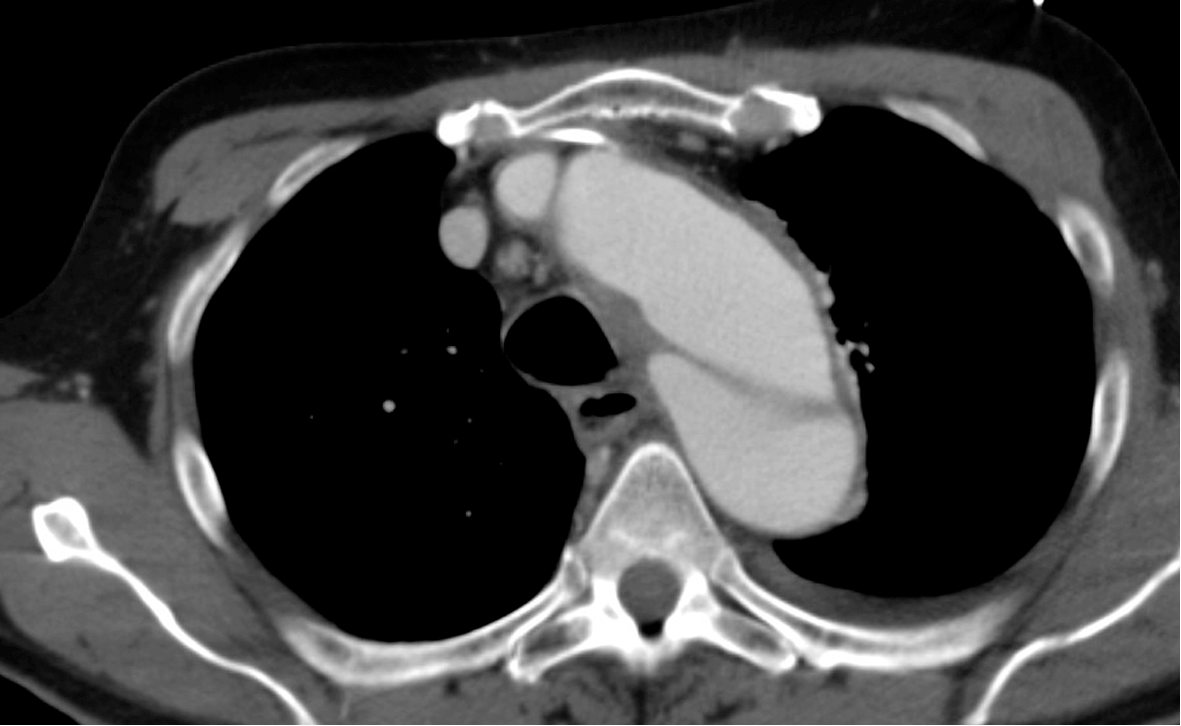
Thoracic aortic CT-scan at admission reveals a classic Stanford type B aortic dissection without clear false lumen dilatation.
